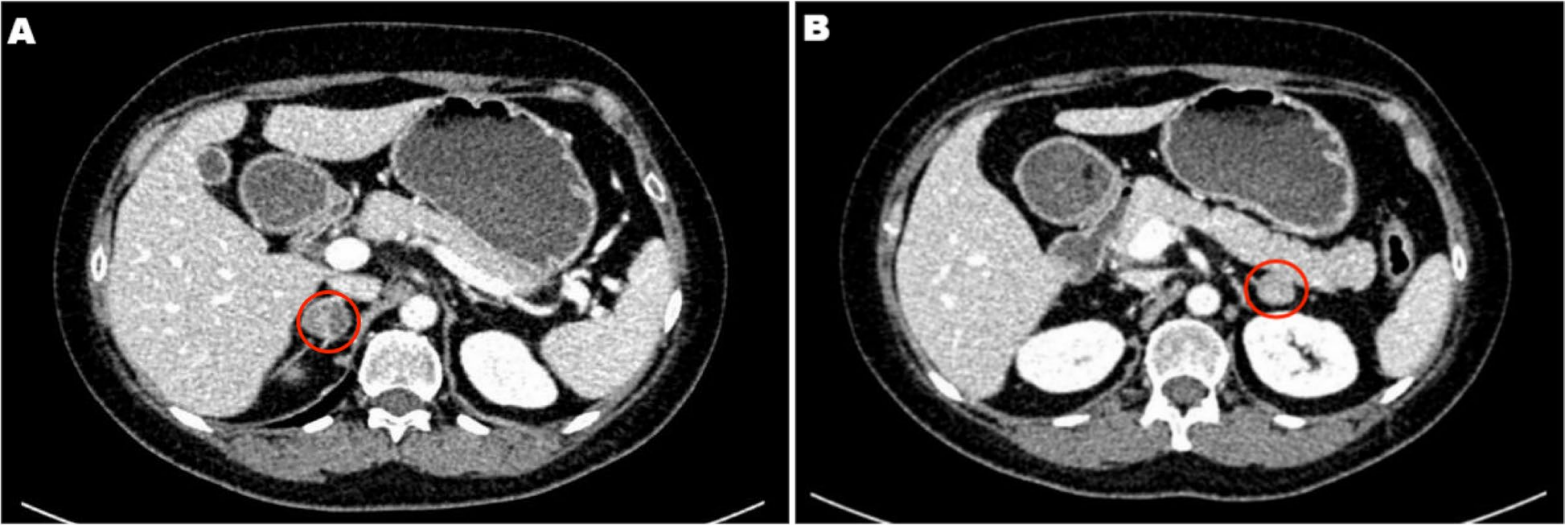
Adrenal CT of the patient: A nodule with a size of approximately 1.6 × 1.5 cm was found in the left adrenal gland, and a nodule with a size of approximately 2.2 × 1.8 cm was found in the right adrenal gland. Irregular mild to moderate enhancement was on enhanced CT, and the surrounding fat gap was clear
